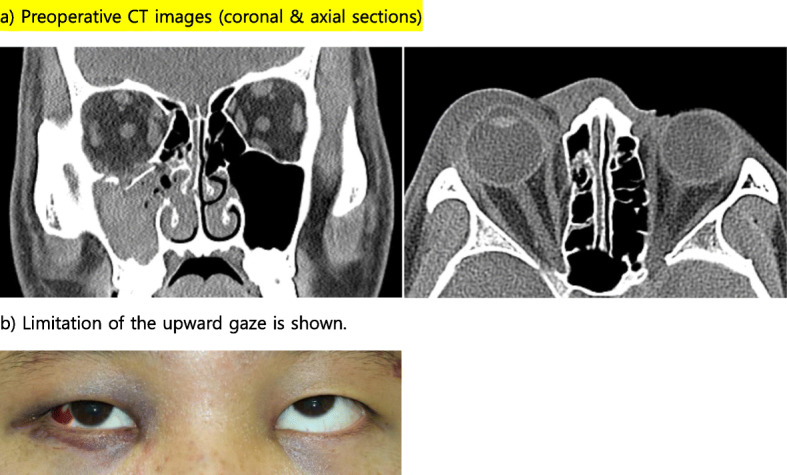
a Preoperative CT images (coronal and axial sections). b Limitation of the upward gaze is shown
